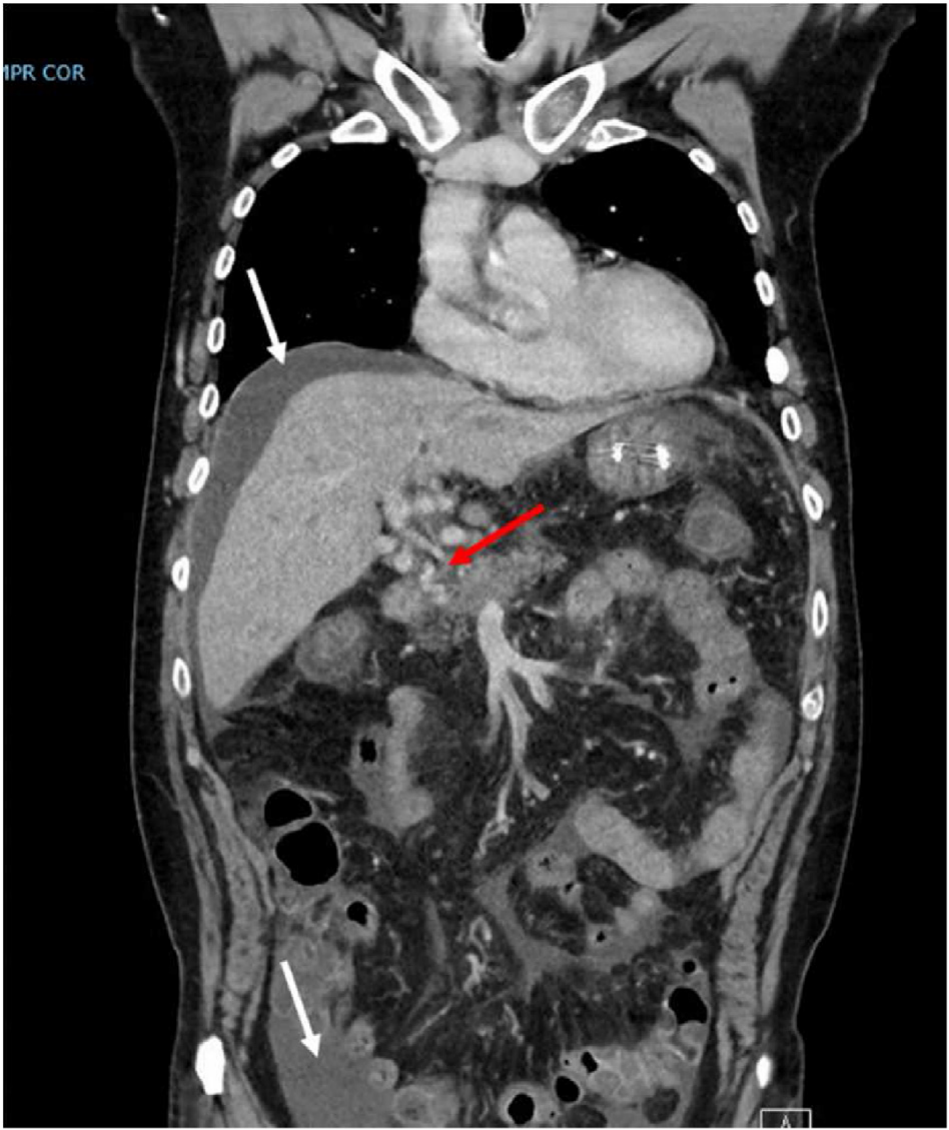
Coronal view of a CT scan at diagnosis showing portal hypertension with extensive collateral veins (red arrow) and ascites (white arrows).
